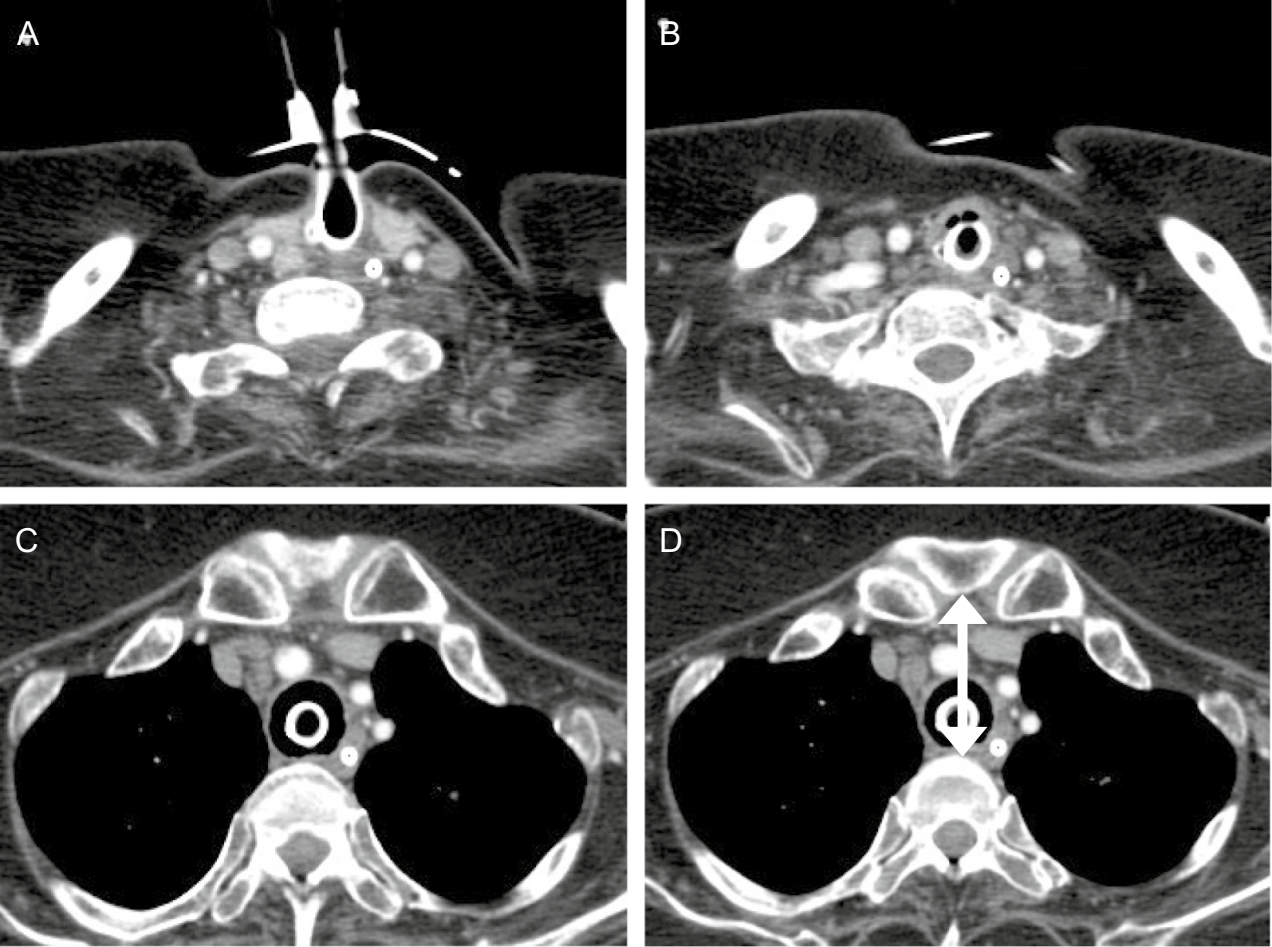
Enhanced computerized tomography (CT) on hospital day 1. A, B Tracheostomy incision was placed at the level of third tracheal ring, and no spinal and arterial deformities were observed. C, D Although the innominate artery was in close proximity of the trachea, no extravasation of contrast media was shown in enhanced CT. D The arrow shows the distance (46 mm) between the posterior surface of the sternum and the anterior surface of the cervical spine
